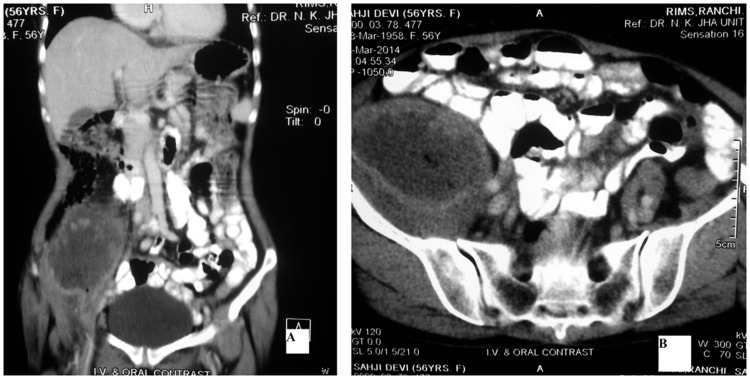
Abdominal computed tomography scans. Coronal view (A) and axial view (B) showing an ill-defined non-enhancing lesion approximately 12.5 × 8.0 × 7.5 cm in size in the right lumbar and iliac region, involving the anterior abdominal wall in its lower part, with air foci within it.
